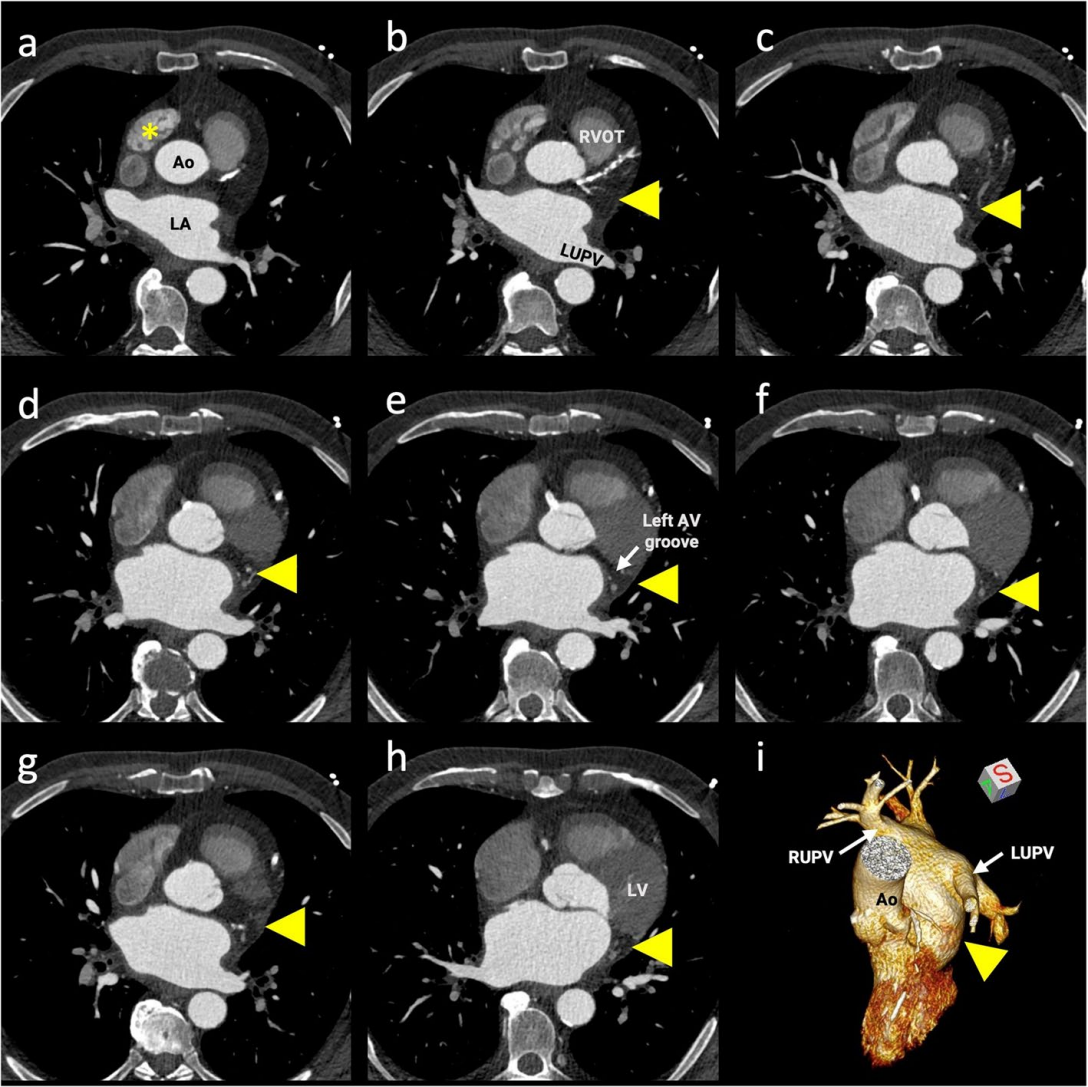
Cardiac contrast-enhanced computed tomography (CCT) images. A–H CCT axial-plane images cranial to caudal. Arrowheads show absence of the left atrial appendage in its normal position (arising from the anterolateral wall of the left atrium [LA] at the level of the left upper pulmonary vein [LUPV] and typically extending anteriorly, overlapping the left border of the pulmonary trunk and the circumflex artery). Asterisks represent the normally developed right atrial appendage. I Three-dimensional volume-rendered reconstruction of a left chamber comprehensively demonstrates the absence of the left atrial appendage (arrowhead). Ao, aorta; RVOT, right ventricular outflow tract; AV, atrioventricular; LV, left ventricle; RUPV, right upper pulmonary vein
